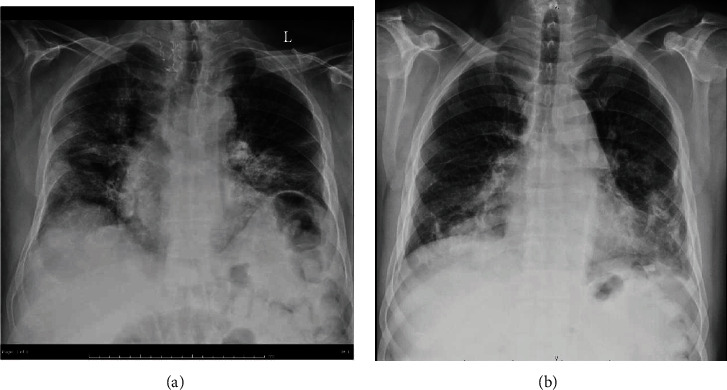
Chest x-rays of two patients (a and b). (a) Focal areas of ground glass opacities and consolidation with reticular interstitial thickening in peripheral and central regions of right upper, bilateral middle, and lower zones. (b) Focal areas of ground glass opacities and consolidation at left middle and bilateral lower lung zones with obliterated left lateral costophrenic recess suggesting pleural effusion.
